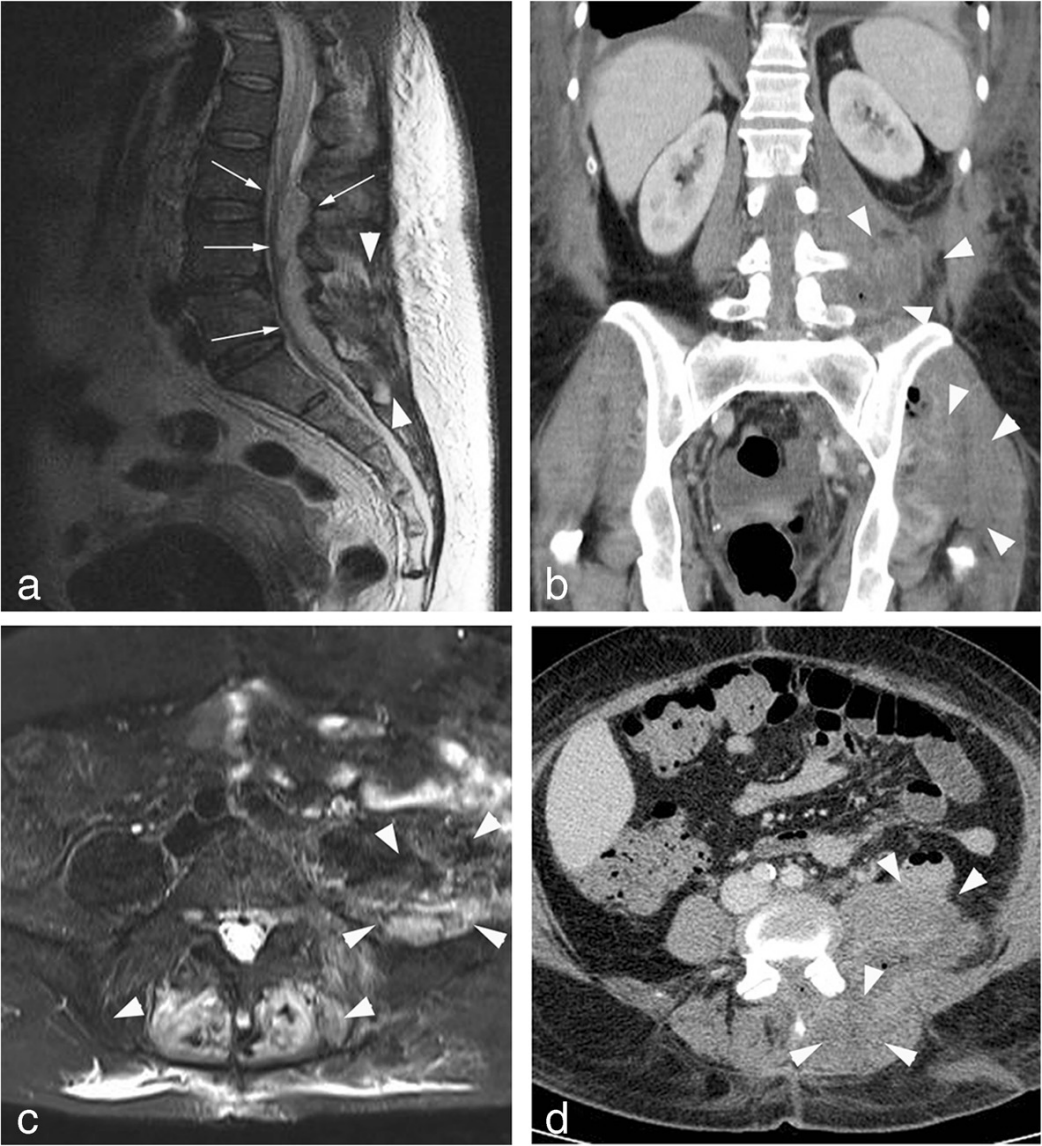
Shows different inflammatory musculoskeletal sites in one patient: lumbar MRI with an epidural abscess (slim white arrows, a) as well as abscesses of the lumbar paravertebral muscles (bold white arrows, a). CT-scan of the abdomen and pelvis and MRI of the spine demonstrating the psoas abscesses on the left side, inflammatory focus in the paravertebral muscles and abscesses in the gluteal muscles on the left side (bold arrows, b, c and d).
